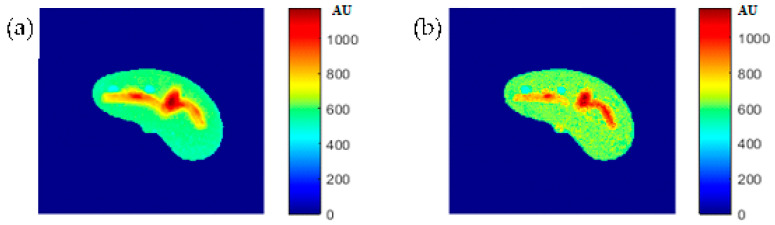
(a) The real MR image with *T_E_* = 10 ms and *T_R_* = 500 ms, and (b) the generated synthetic MR image using the same *T_E_* and *T_R_* values.
